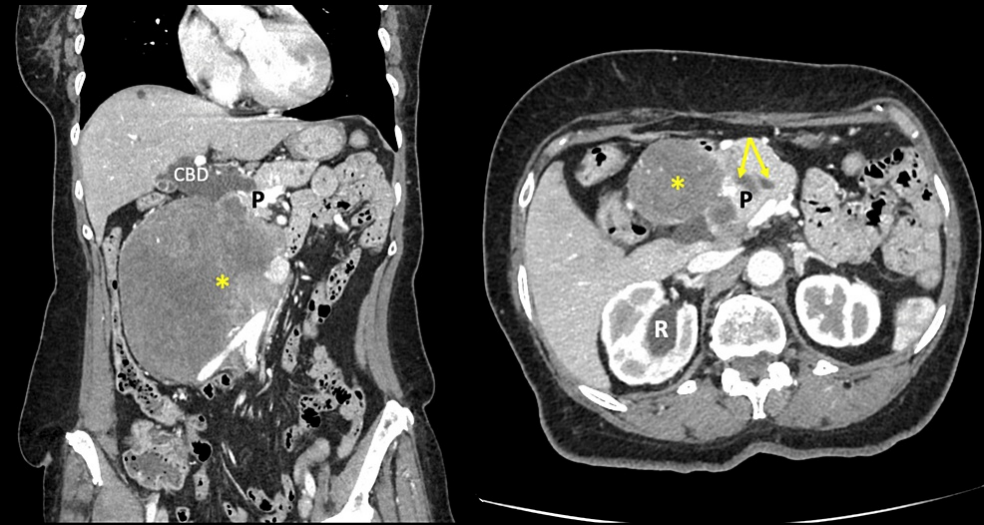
A contrast-enhanced CT scan of the abdomen in coronal (left) and corresponding axial (right) views. The large tumor (asterix) appears intimately related to the pancreatic head and uncinate process (P). In the axial views, a double duct sign (arrows) can be seen. The common duct is markedly dilated (CBD). There is also right hydronephrosis (R) on the axial images due to obstruction by the mass.
